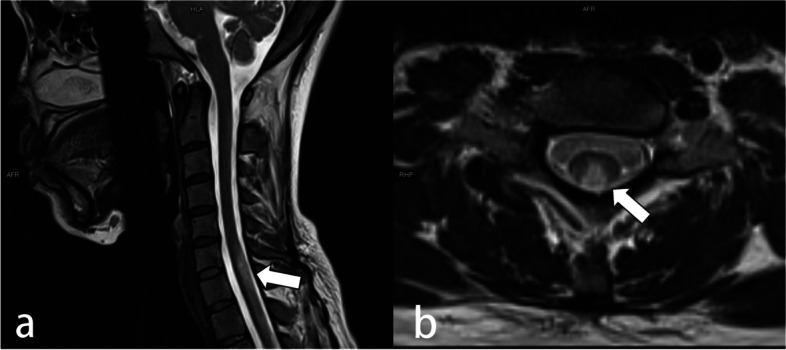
A Sagittal T2-weighted image showing abnormal hyperintense signal in the posterior aspect of the spinal cord (arrow) extending from the level of C6. B Axial T2-weighted image of the cervical spinal cord showing symmetric bilateral hyperintensity in the dorsal and lateral columns(inverted V sign) (indicated by white arrow)
